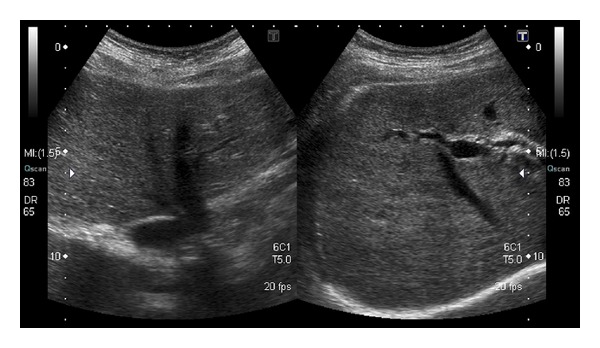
Abdominal ultrasonography (coarsening in liver parenchyma and minimally prominent intrahepatic bile ducts).
